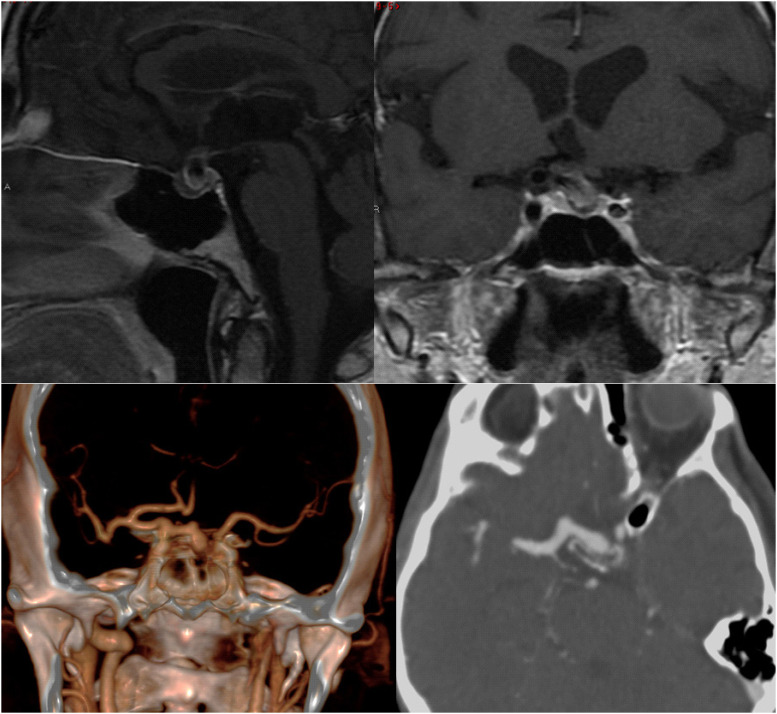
The two images on the top show a pituitary magnetic resonance image of a 10 × 11 × 8 mm sellar mass, highly suggestive of a vascular structure extending from the right internal carotid artery into the sellar region and compressing the pituitary gland. The two images on the bottom show a computed tomography angiography of the cerebral arteries revealing unruptured, partially thrombosed aneurysm of the ophthalmic segment of the right ICA, extending into the sellar region and compressing the pituitary gland.
